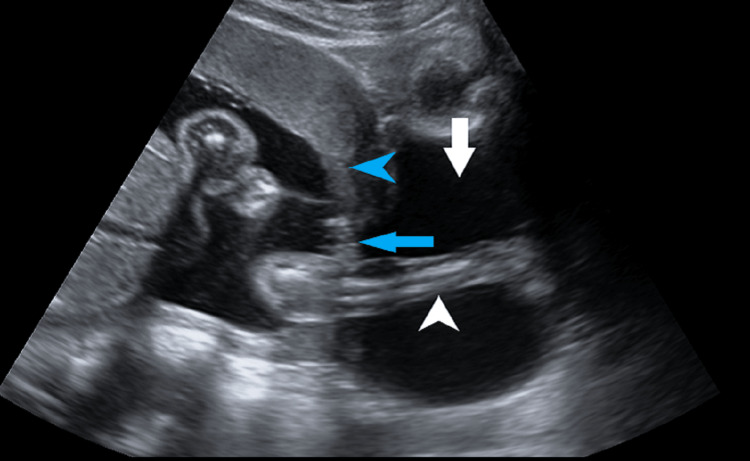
Transabdominal ultrasound image of the sagittal section showing an open U-shaped internal os (blue arrow) and a dilated cervix (white arrow) with fetal membranes and parts (feet) within (white arrowhead). The placenta is anterior, grade 1, with a lower margin reaching up to the internal os (blue arrowhead) suggesting placenta previa grade 3. os: orifice.
